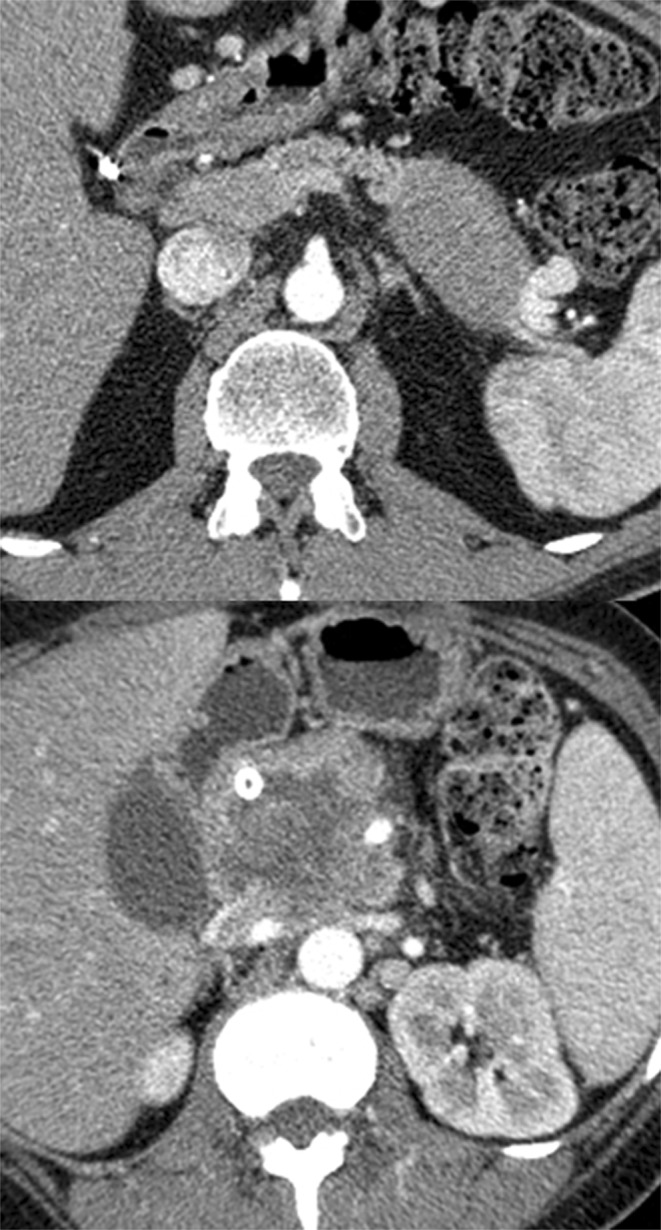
(a,b) neuroendocrine pancreatic tumor with sharp (a) or irregular (b) margins at CT.
